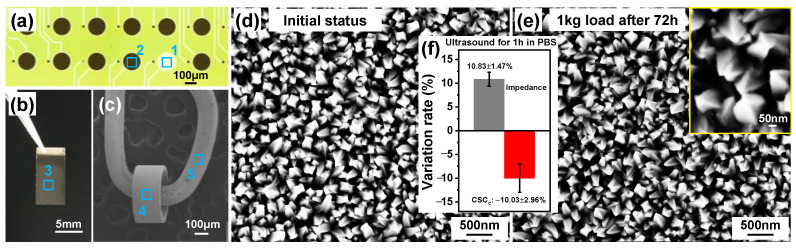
Batch production of Pt nanocones. Pt nanocone array electrodeposited on (a) electrophysiological fMEA, (b) platinized silicon wafers, and (c) Pt wire and cochlear implant. The numbers marked on panels (a–c) were chosen to observe the morphology, with point 1 as the unmodified electrode for reference. (d) The initial status and (e) after 1 kg load for 72 h of a Pt−nanocone−array−based platinized silicon wafer, the inset in (e) shows the enlarged image of Pt nanocone with excellent stability. (f) The variation rate of impedance and CSC_c_ after ultrasonic tests for 1 h in PBS.
